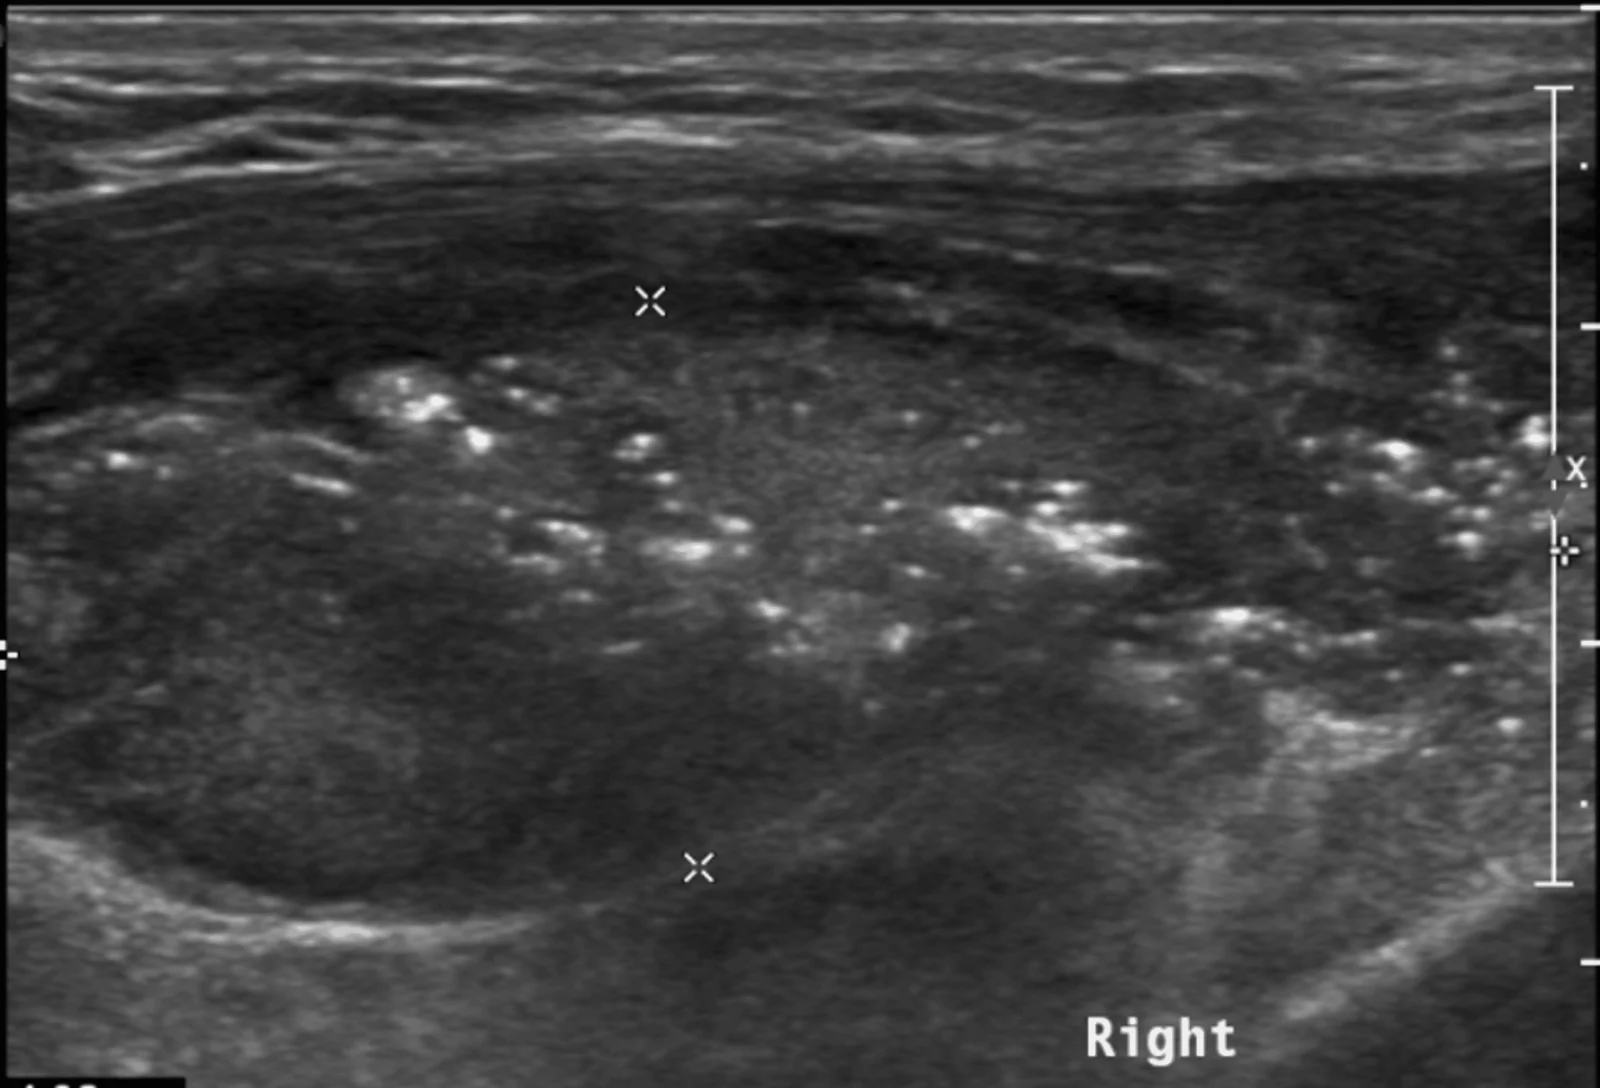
Ultrasound of the right chest mass Multiple internal echogenic foci producing posterior dirty shadowing, compatible with intralesional gas
